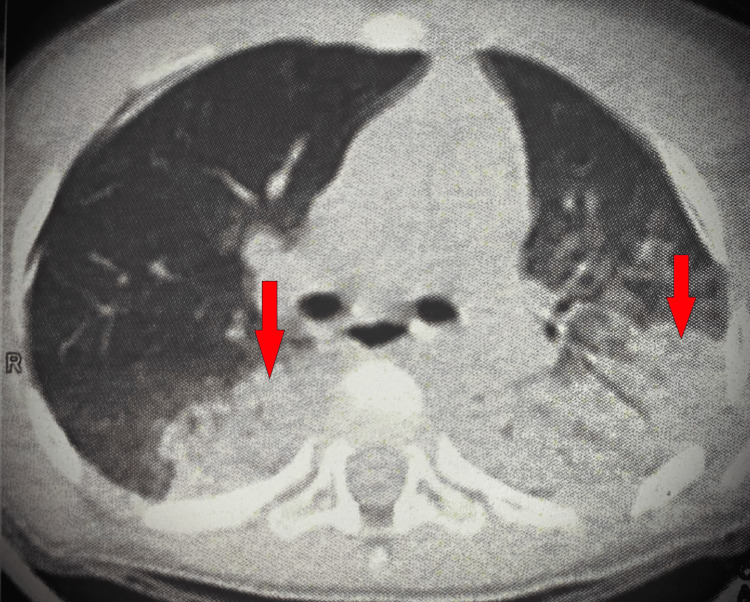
Chest CT showed bilateral lung opacities (arrows show examples of the opacities)
